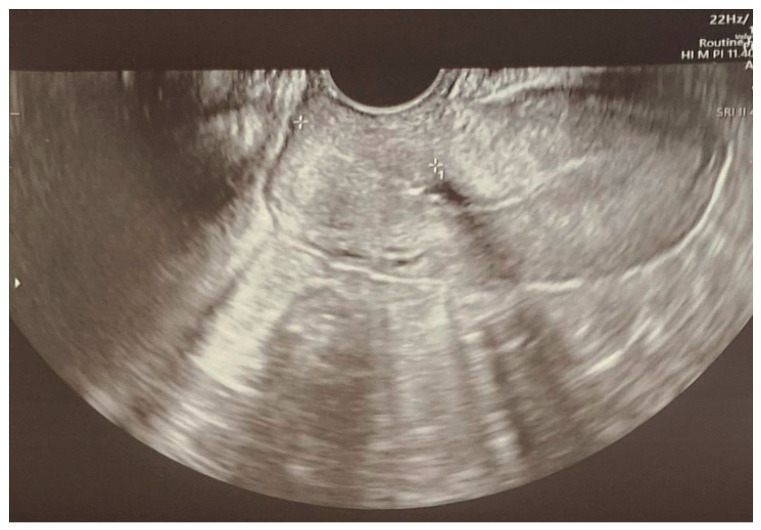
Transvaginal ultrasound revealed correct uterus healing 3 months after the cesarean section.
